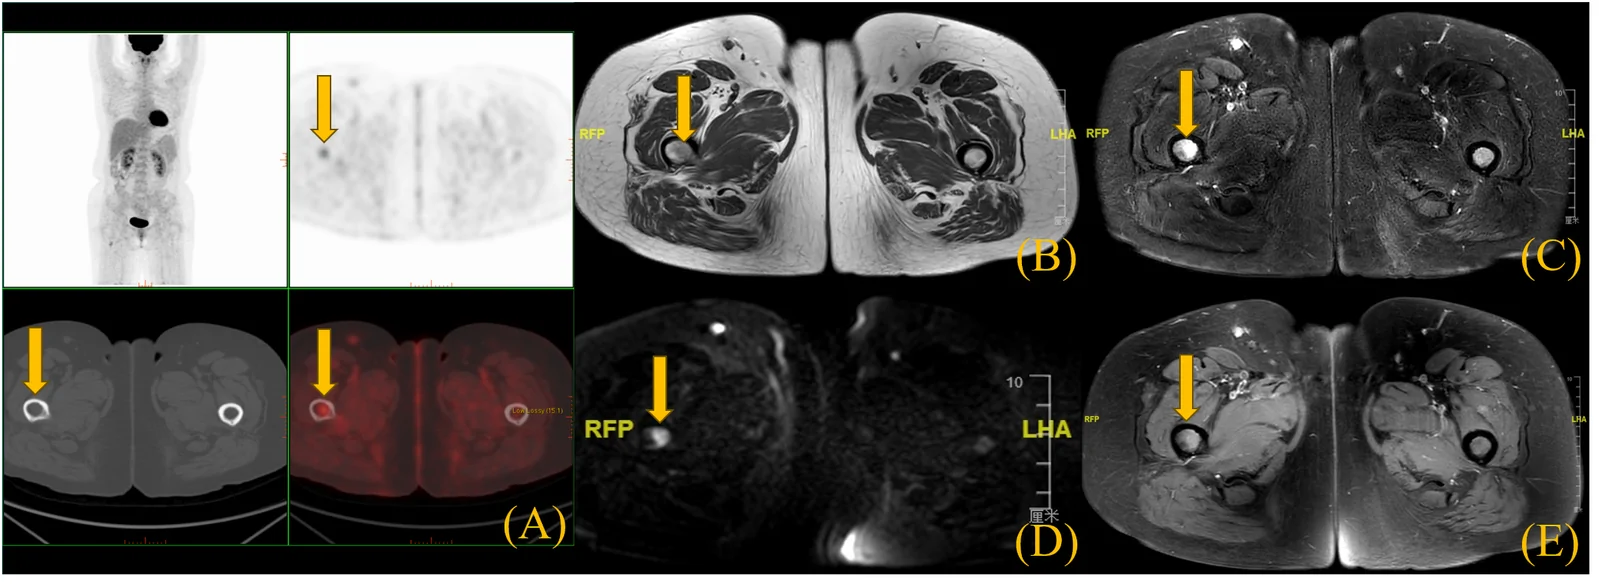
A 51-year-old female patient was diagnosed with poorly differentiated squamous cell carcinoma of the nasopharynx by nasopharyngeal endoscopy. ¹^8^F-FDG PET-CT (A) revealed a nodular focus of increased FDG uptake in the bone marrow cavity of the right upper femur with SUVmax = 3.21. The density of the bone marrow cavity was slightly higher compared to the contralateral side. A physician with nearly 20 years of experience in ¹^8^F-FDG PET-CT interpretation considered the lesion likely benign at the time of examination. On T1WI (B), the lesion showed slightly hypointense signal; on T2WI (C) and DWI (D), it appeared hyperintense; and mild enhancement was observed after contrast administration (E). Another radiologist with approximately 20 years of imaging experience regarded the lesion as metastatic. The MLP model (Deep + Radiomics(T) + clinical features + SUVmax) calculated the probability of distant metastasis in this patient as 0.9082629675, further supporting that our model provides auxiliary reference for preliminary risk screening of SDM.
